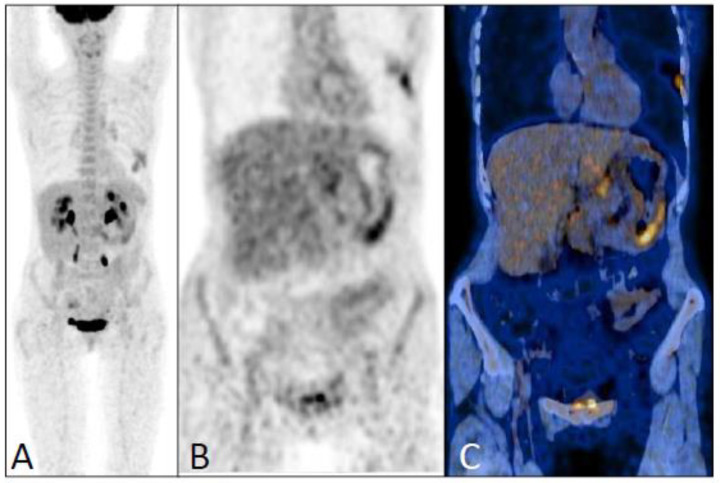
An FDG-PET/CT scan performed one year after the initial diagnosis of ILC (pT1bN0, SBR II, Ki67 = 30%) in a 56-year-old patient treated by aromatase inhibitor, due to a pyloric ulcer. Low increased FDG uptake in the thickened gastric wall was demonstrated on the maximum intensity projection (A), coronal PET (B), and PET/CT fusion (C) images. The increased uptake in the left lower chest was related to a pneumonitis. This patient died nine months later from disease progression of gastric adenocarcinoma.
